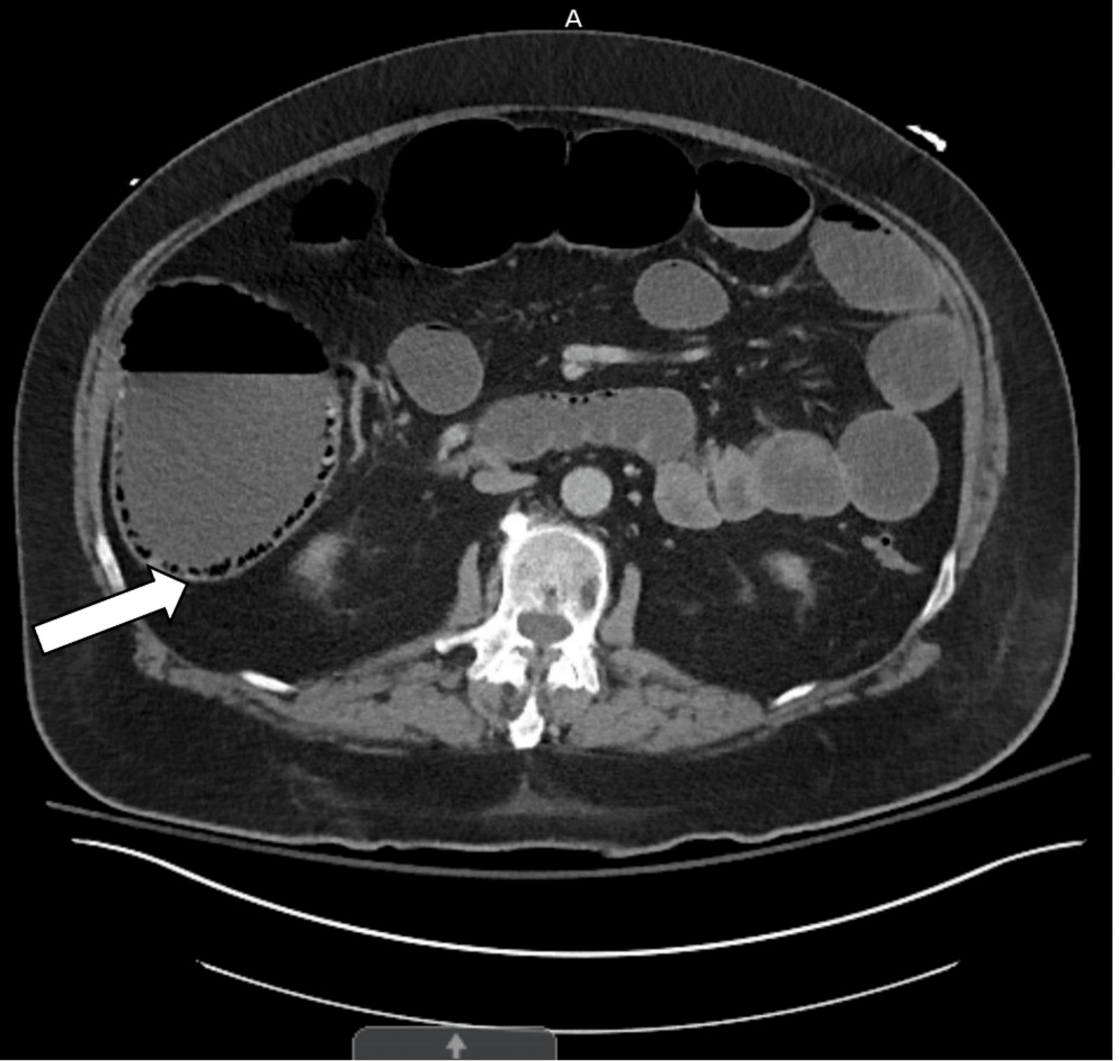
A CT scan of the abdomen and pelvis with IV contrast showed dilated ascending colon with circumferential pneumatosis intestinalis (arrow).
